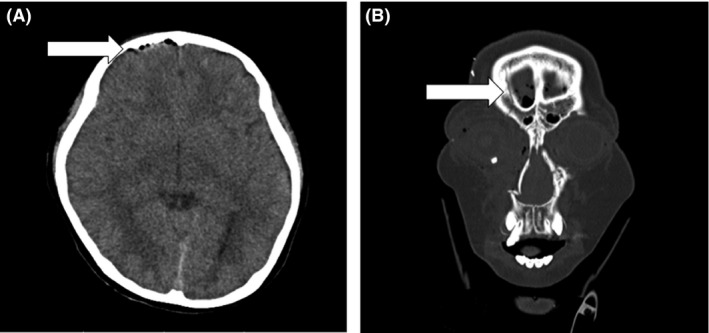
Computed tomography of a 9‐year‐old boy who was injured in a car accident, carried out on arrival at hospital. A, Pneumocephalus inside the frontal cranial bone (arrow). B, Fracture of the frontal cranial bone (arrow).
